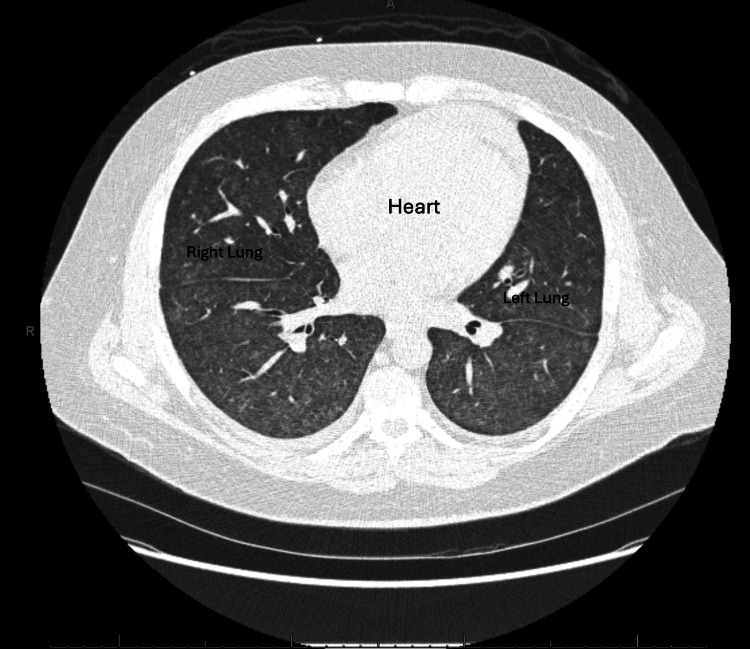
Axial view of the CT scan of the chest shows bilateral ill-defined ground-glass opacities.
